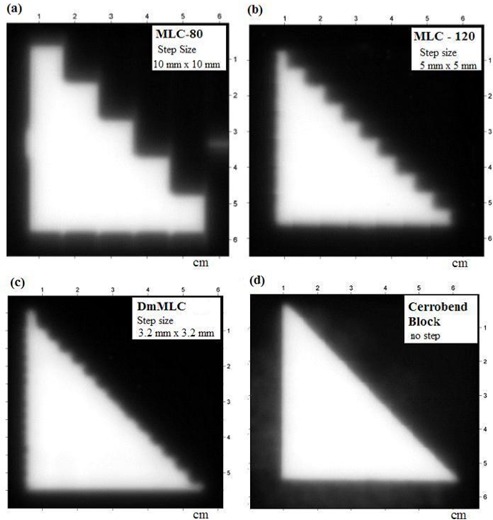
Radiographic films irradiated with 6 MV X‐rays. (a) Multileaf collimator (MLC)–80 with 1‐cm leaf width. (b) MLC‐120 with 0.5‐cm leaf width. (c) Dual‐layer micro‐MLC with 0.32‐cm leaf width. (d) Cerrobend (Cerro Metal Products, Bellefonte, PA) blocks.
